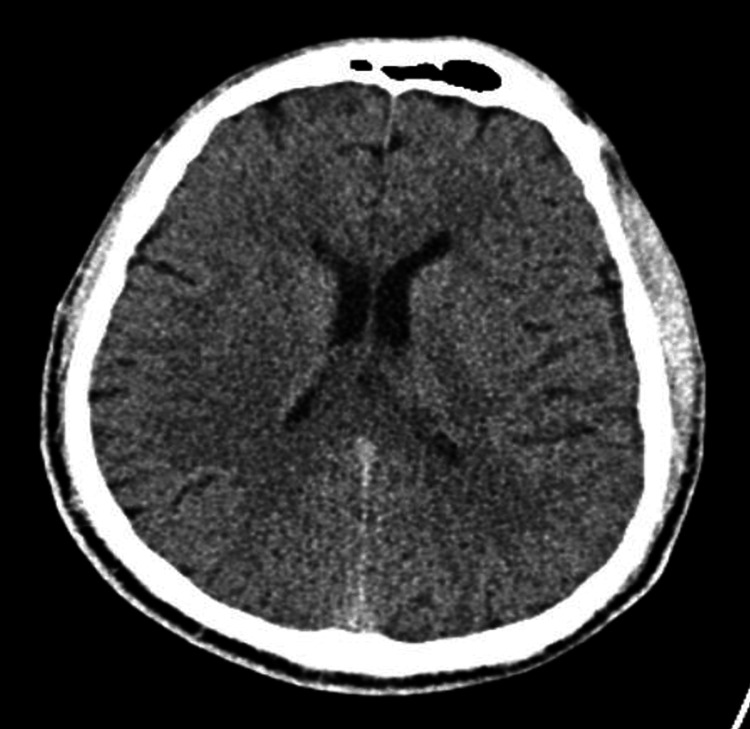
Non-contrast computed tomography of the brain showing no acute intracranial pathology.
